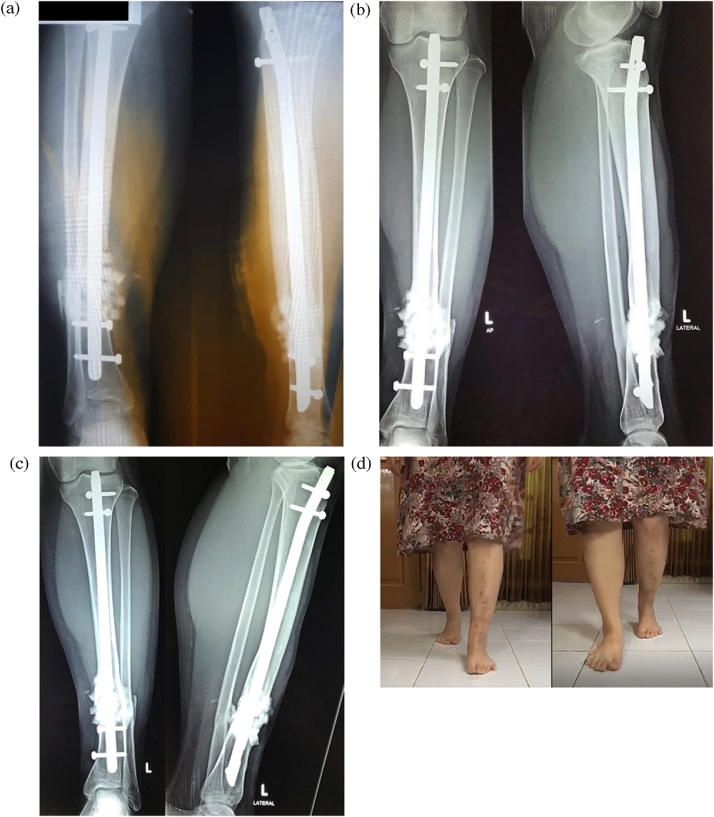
(a) Post-operative x-ray, (b) two months after surgery, (c) four months after surgery, (d) the patient was pain-free and able to walk.
